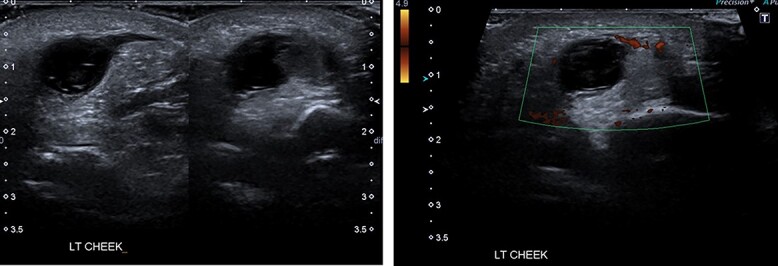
Ultrasound scan images of the lesion the left cheek, showing fluid and solid soft tissue suggestive of haematoma.
